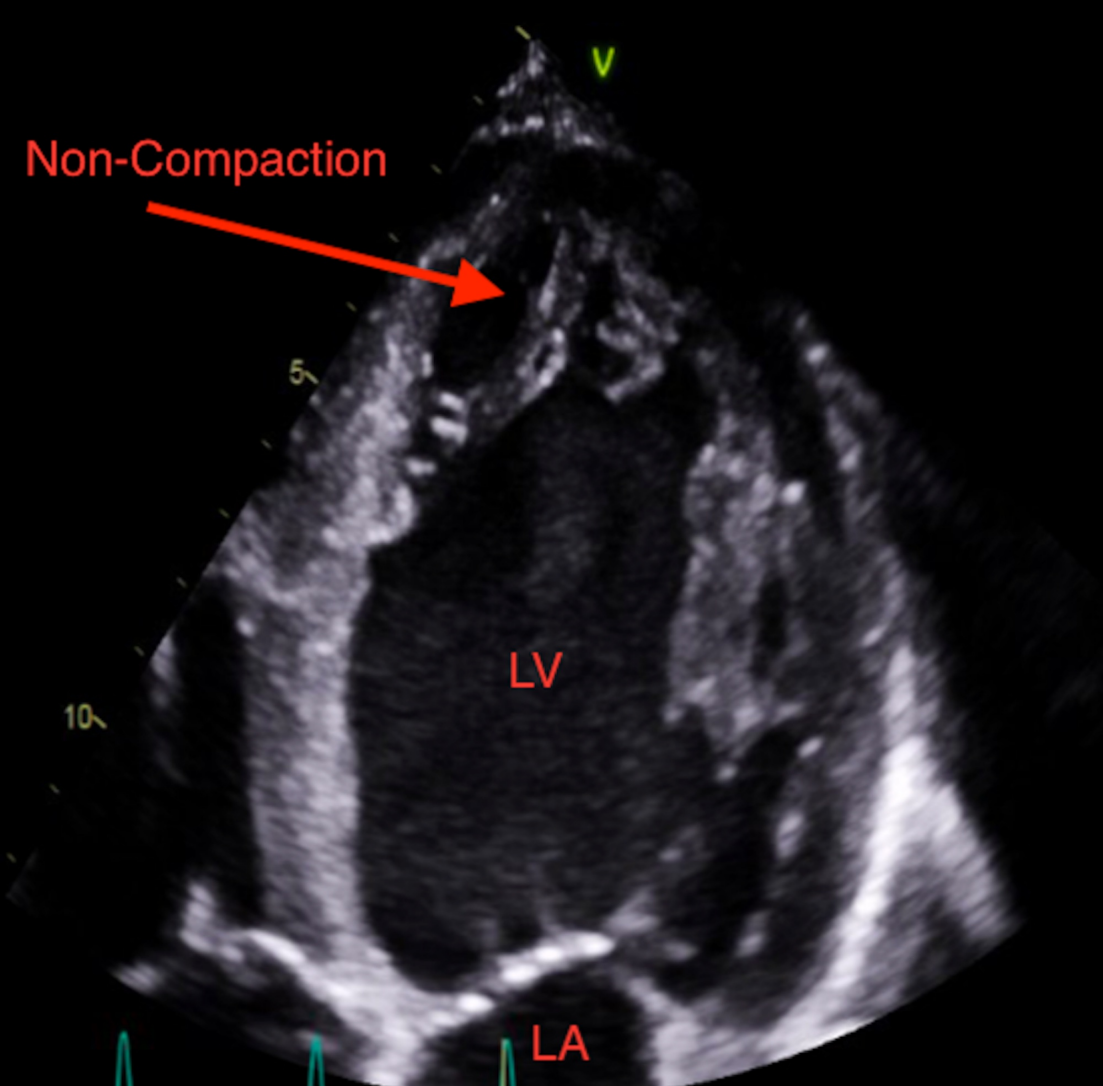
Apical 2 chamber view showing LVNC (left ventricular non-compaction). LV: Left ventricle; LA: Left atrium.
